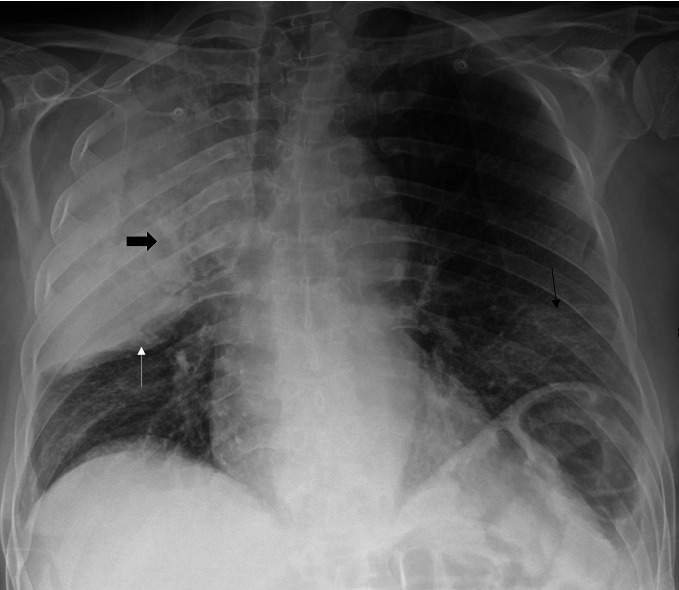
Chest X-ray. AP projection. Consolidation expanded the right upper lobe, with a mildly bulging horizontal fissure and a thin white arrow. Several small foci of cavitation exist within the consolidated RUL, one of which is indicated by the thick black arrow. Bilateral lower zone ground-glass opacities, thin black arrow on the left.
